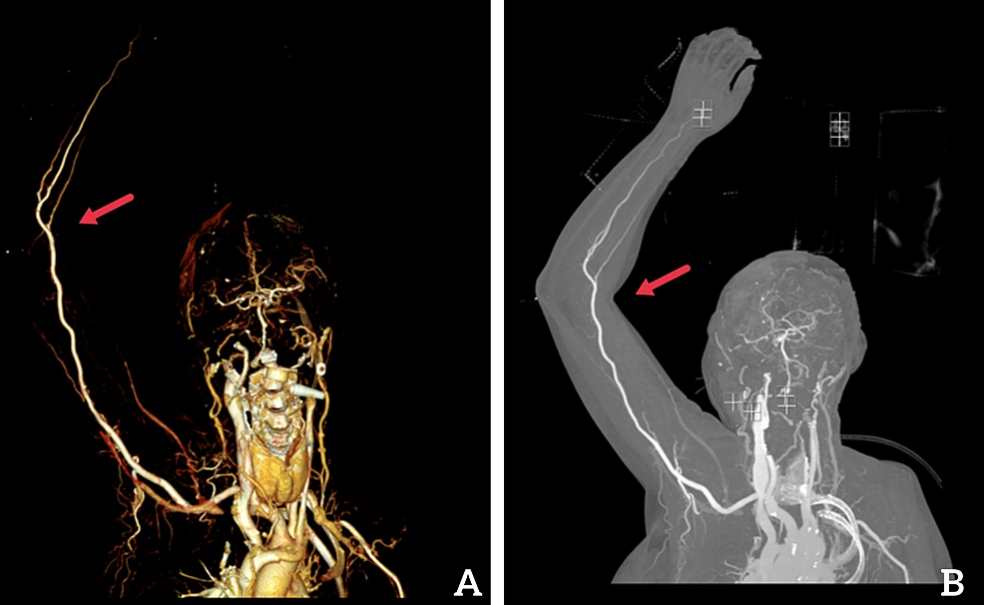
Computed tomography angiogram (CTA) of right upper extremity: (A) three-dimensional reconstruction view, (B) coronal reconstruction view. Red arrow indicates the location of the hematoma.
